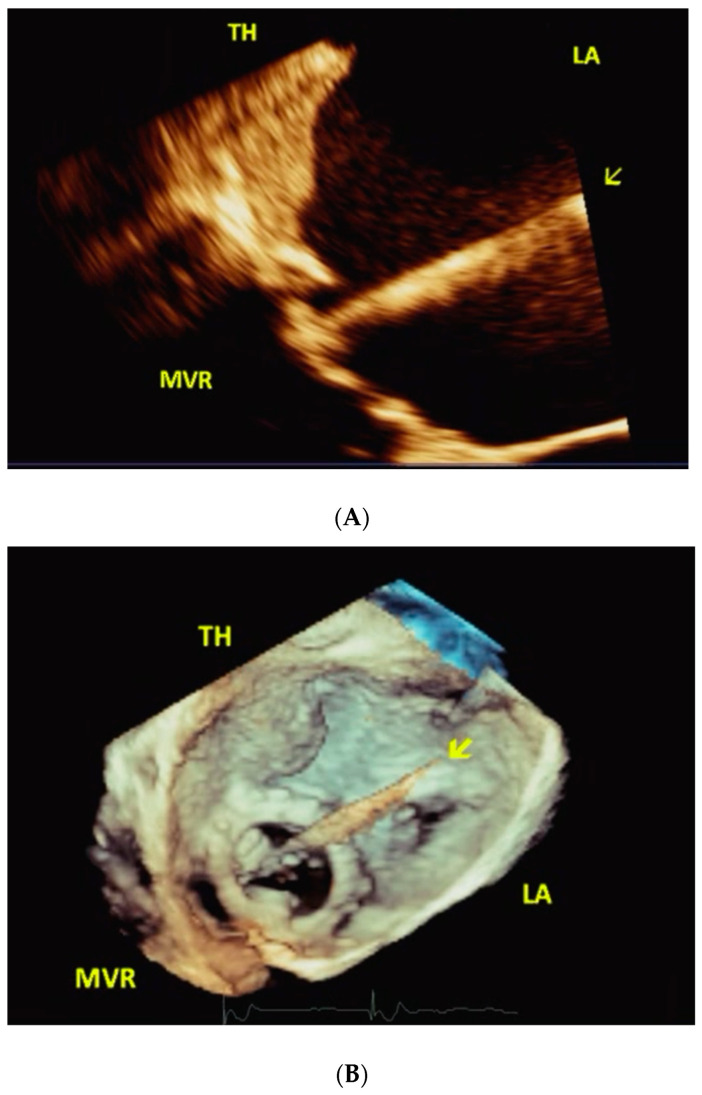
(A,B). 67-year-old male with severe MVR stenosis (Case 4). During procedure. Live/real-time three-dimensional transesophageal echocardiography (A,B). The arrow points to the catheter deployment device passing through MVR and bypassing the TH in MPR mode in A and in full volume mode in B Abbreviations as in previous Figures.
